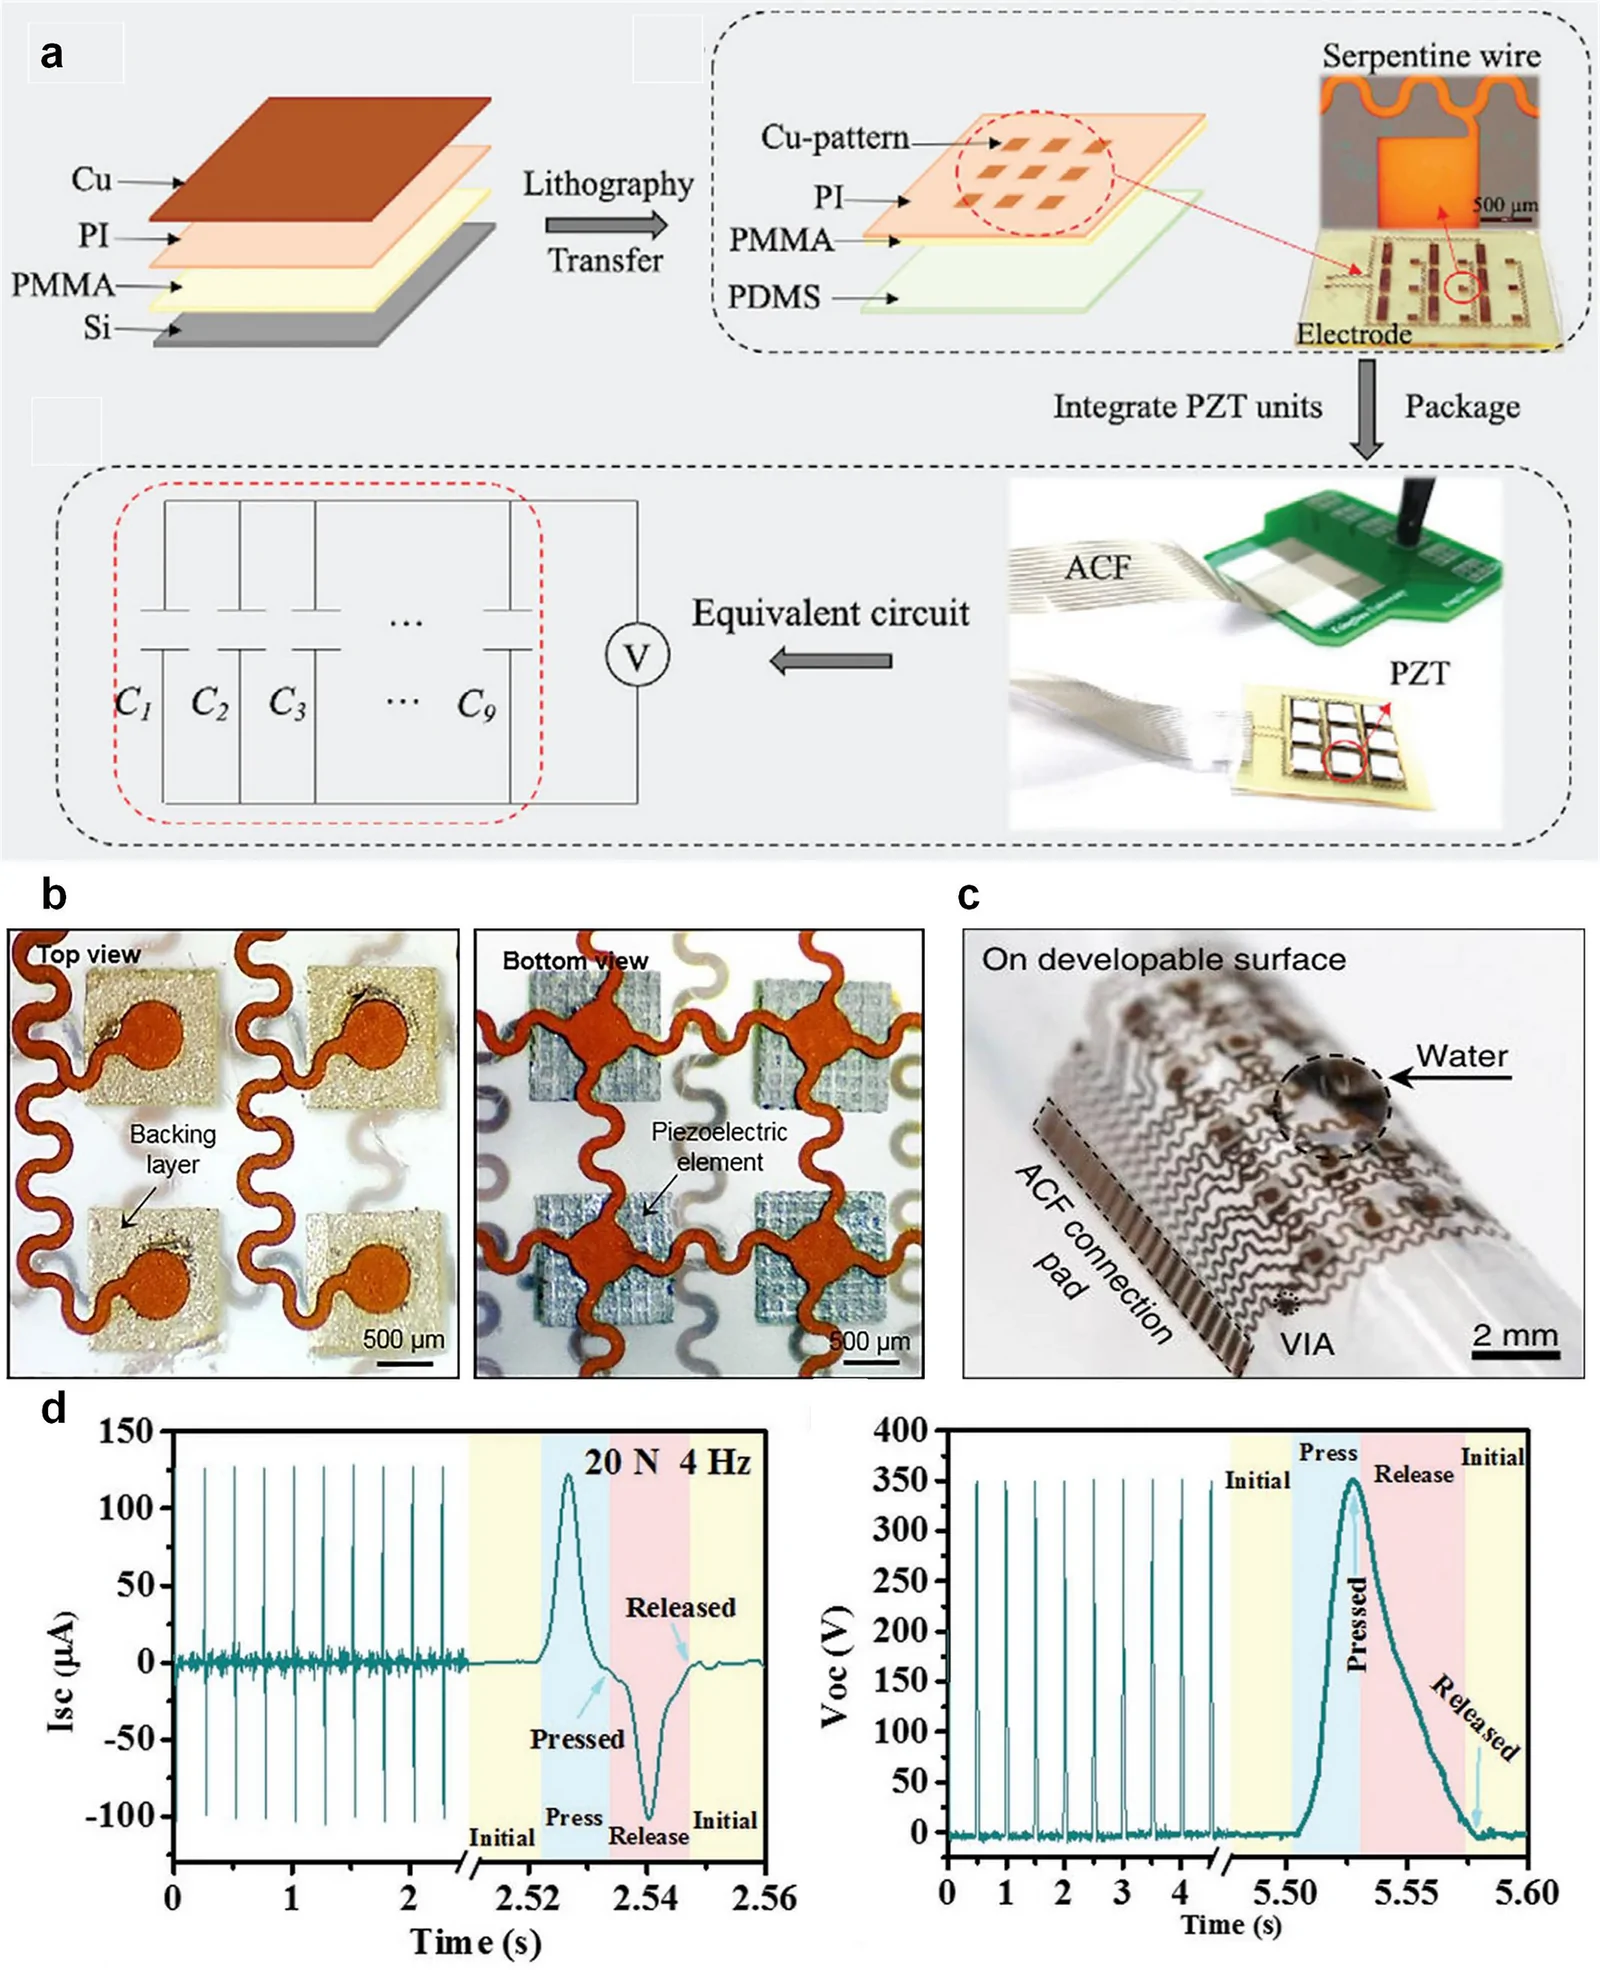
a PMMA, PI and Cu metal layers are spin-coated on the Si substrate. Lithography, etching and transfer printing are used to fabricate the flexible circuit. Piezoelectric ultrasonic units (PZT—4) are integrated into the flexible circuit, which is then packaged with a thin (≈200 μ hydrogel patch. Reproduced with permission [90]. Copyright 2021, Wiley–VCH GmbH. b Optical image (top view) of four elements, showing the morphology of the backing layers and top electrodes, and the optical image (bottom view) of four elements, showing the morphology of the piezoelectric material and bottom electrodes. Reproduced with permission [34]. Copyright 2018, AAAS. c Bent around a developable surface. Reproduced with permission [31]. Copyright 2018, Springer Nature. d Compression test of the hybrid NG: short circuit current and open circuit voltage. Reproduced with permission [118]. Copyright 2020, WILEY–VCH Verlag GmbH & Co. KGaA
